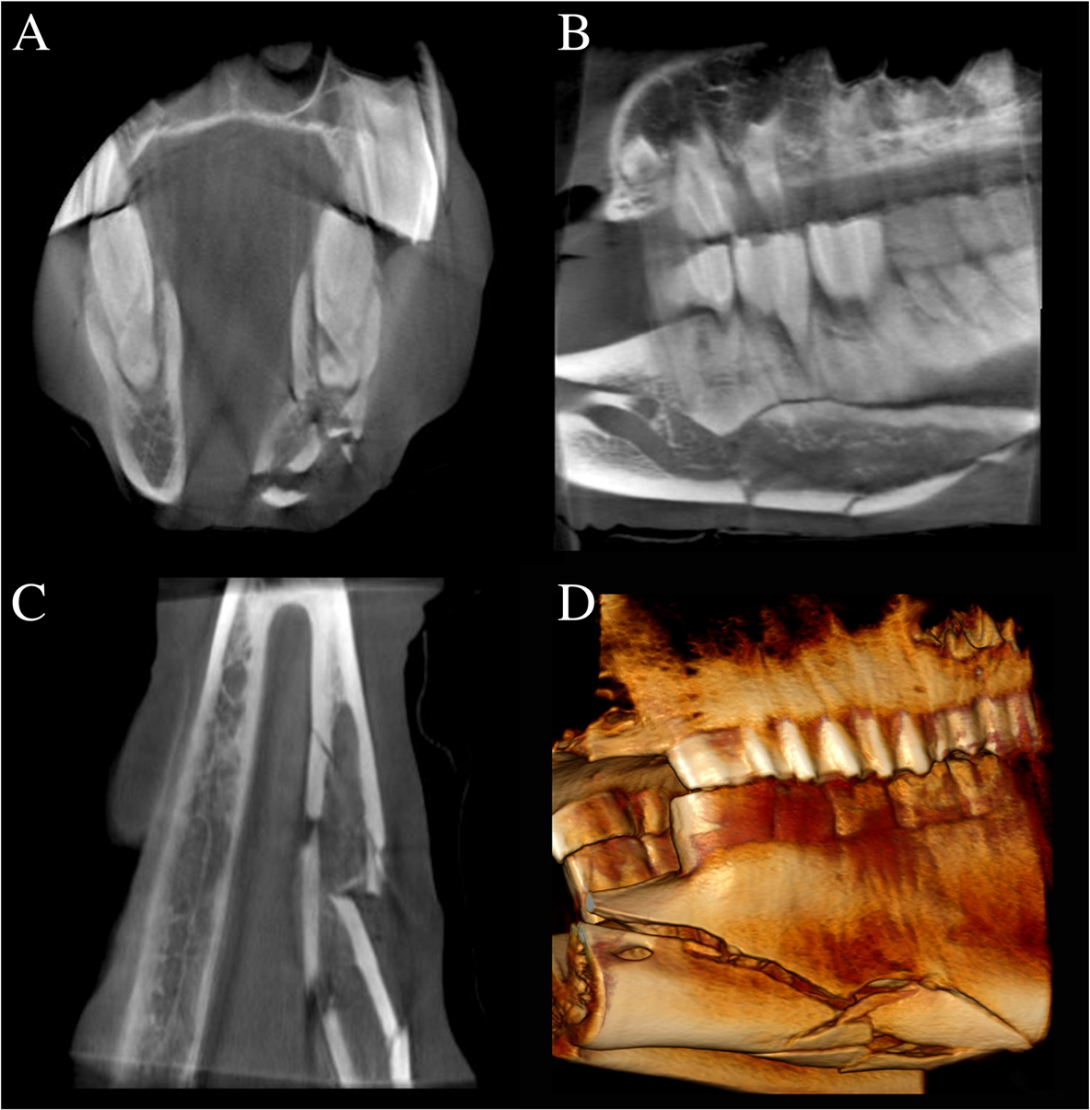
CBCT scan of a 20-year-old Akhal-Teke/Lusitano mix with a left comminuted mandibular fracture. a-d Transverse, sagittal and dorsal reconstruction and 3D volume rendering showing a left comminuted mandibular fracture in the diastema
